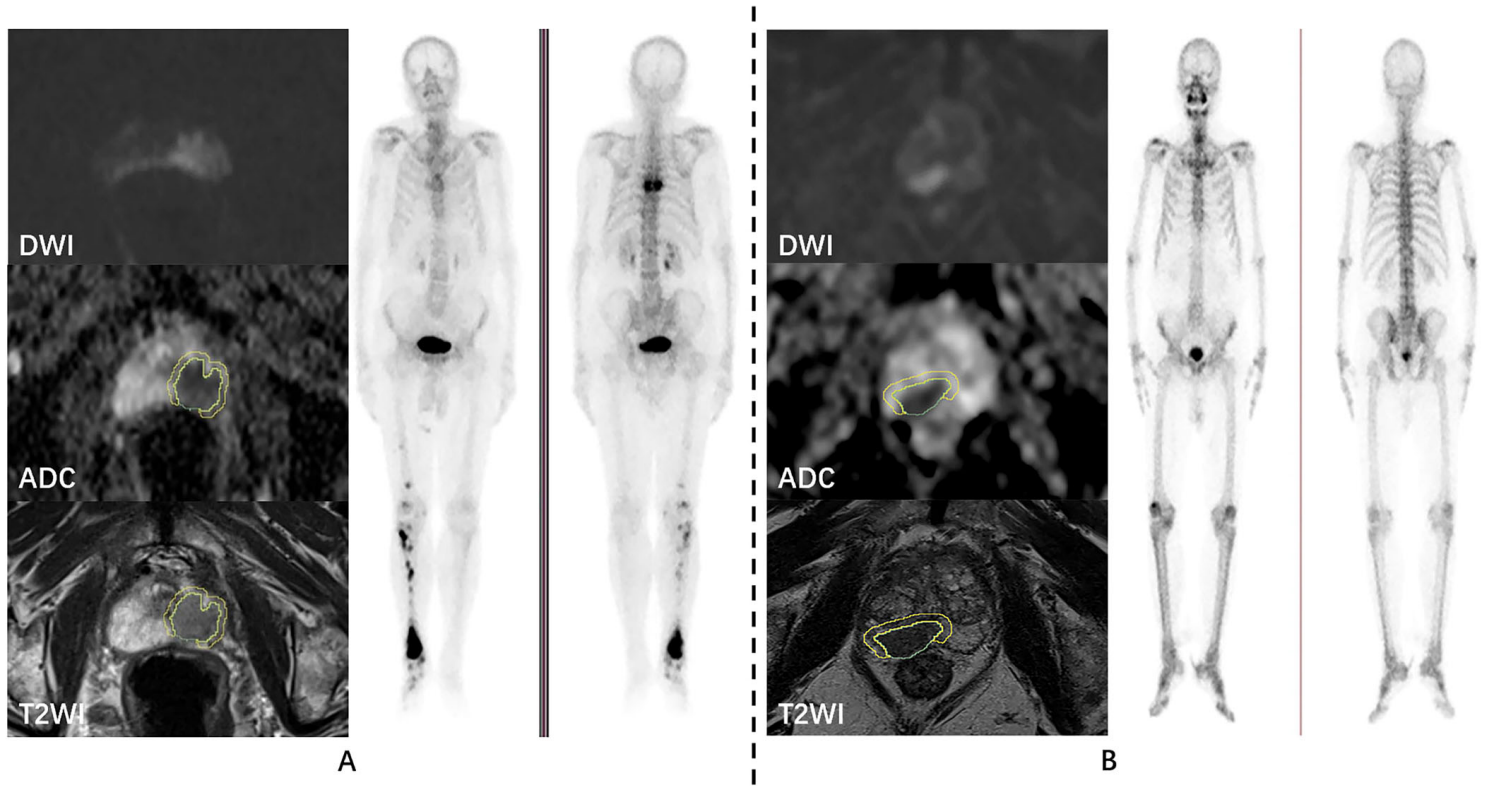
Examples of prostate cancer patients. DWI, ADC, T2WI, and whole-body skeletal imaging images of prostate cancer patients with bone metastasis (A) and without bone metastasis (B), respectively.
